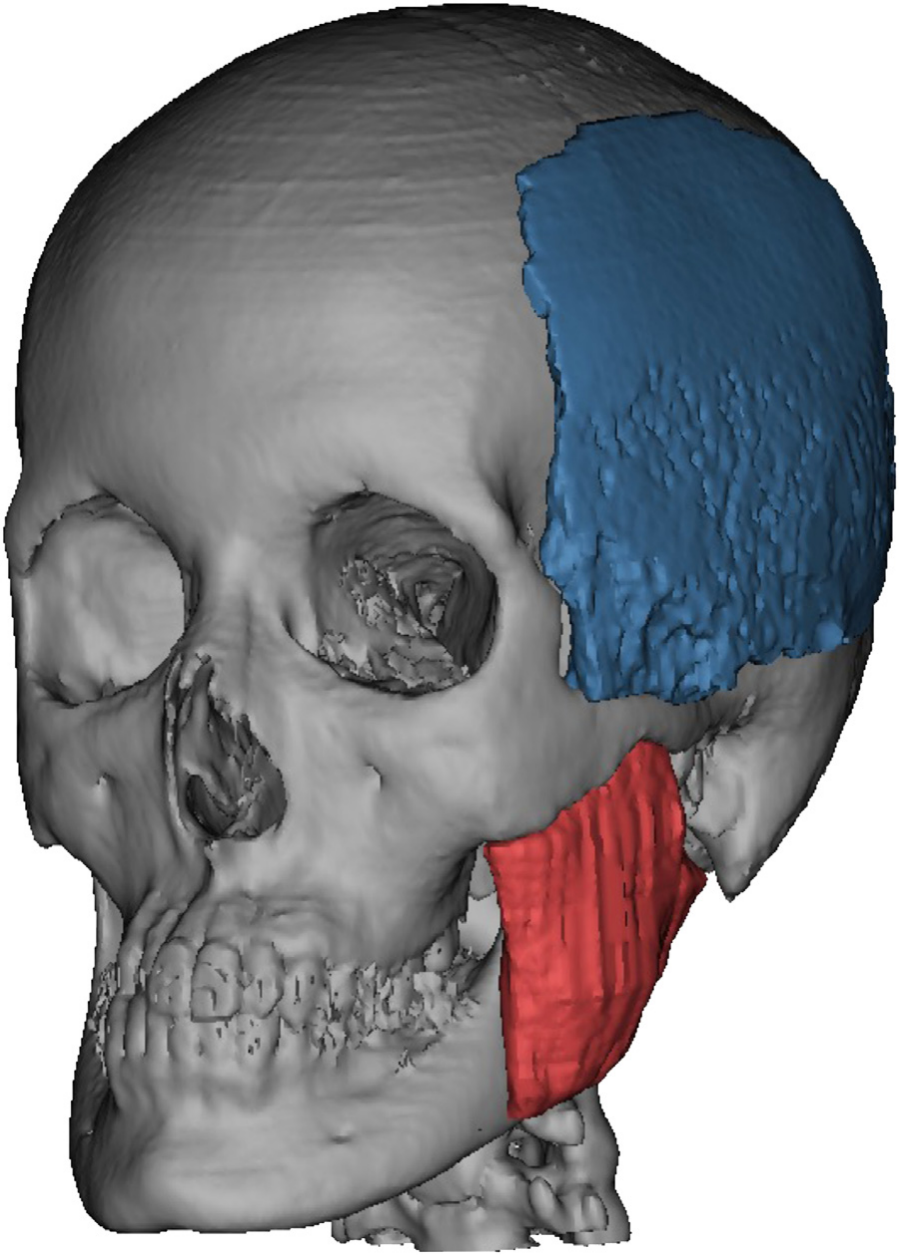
Measurement of temporal and masseter muscle volumes. The 3D temporal and masseter muscles were constructed from the CT data, and the volume was measured. Statistical analyses were performed to evaluate differences in the temporal muscle volume in the left and right muscles (variable temporal muscle volume = right temporal muscle volume - left temporal muscle volume).
